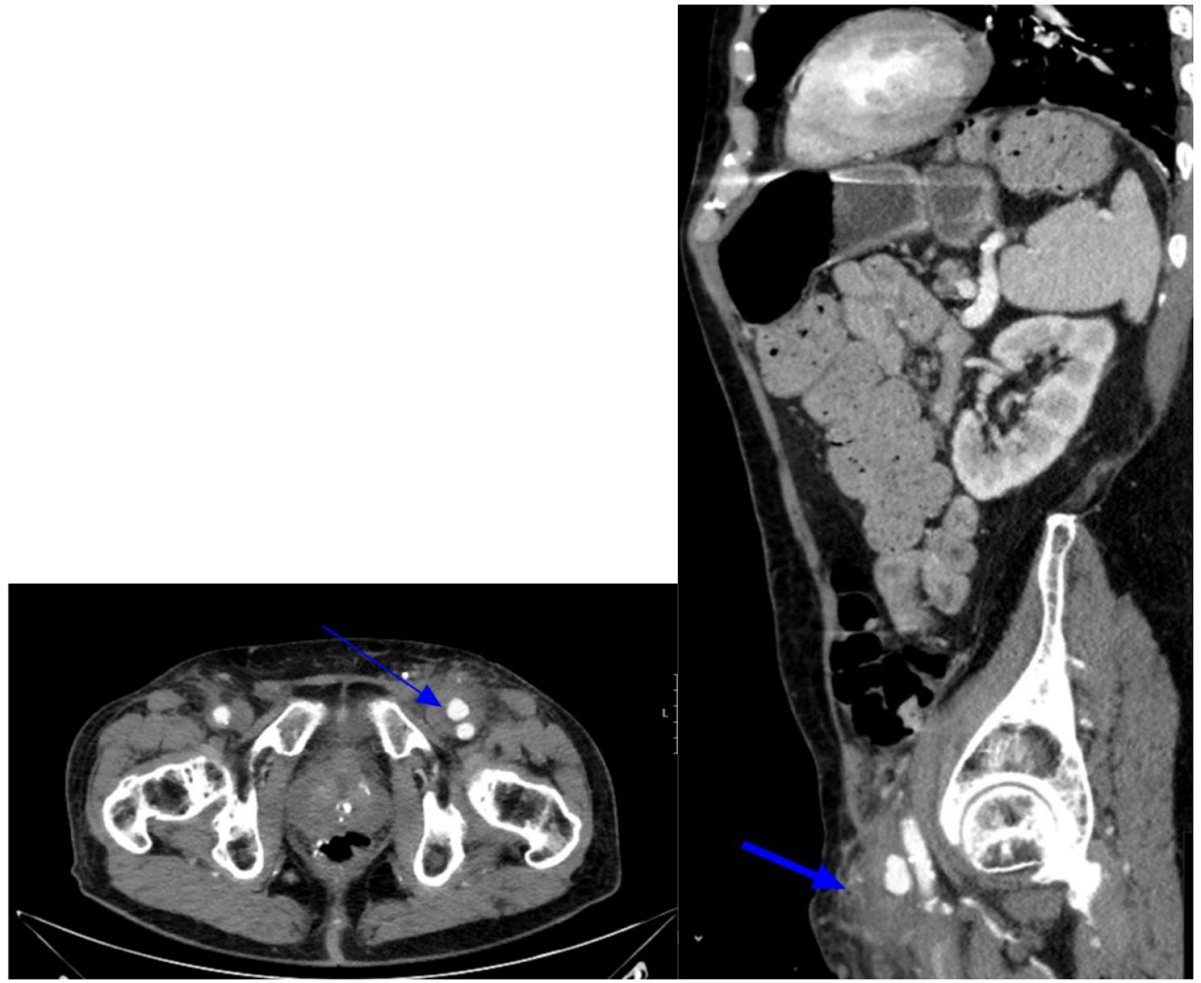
88 year old male with history of cerebral vascular accident presenting with nausea and vomiting. CT abdomen and pelvis in soft tissue window in Axial (A) and sagittal (B) reformats demonstrate a 1.4 cm hyperdensity (blue arrow) anteriorly at the left common femoral artery, most consistent with a pseudoaneurysm and heterogeneous density surrounding left common femoral vessels, suggestive of hematoma
